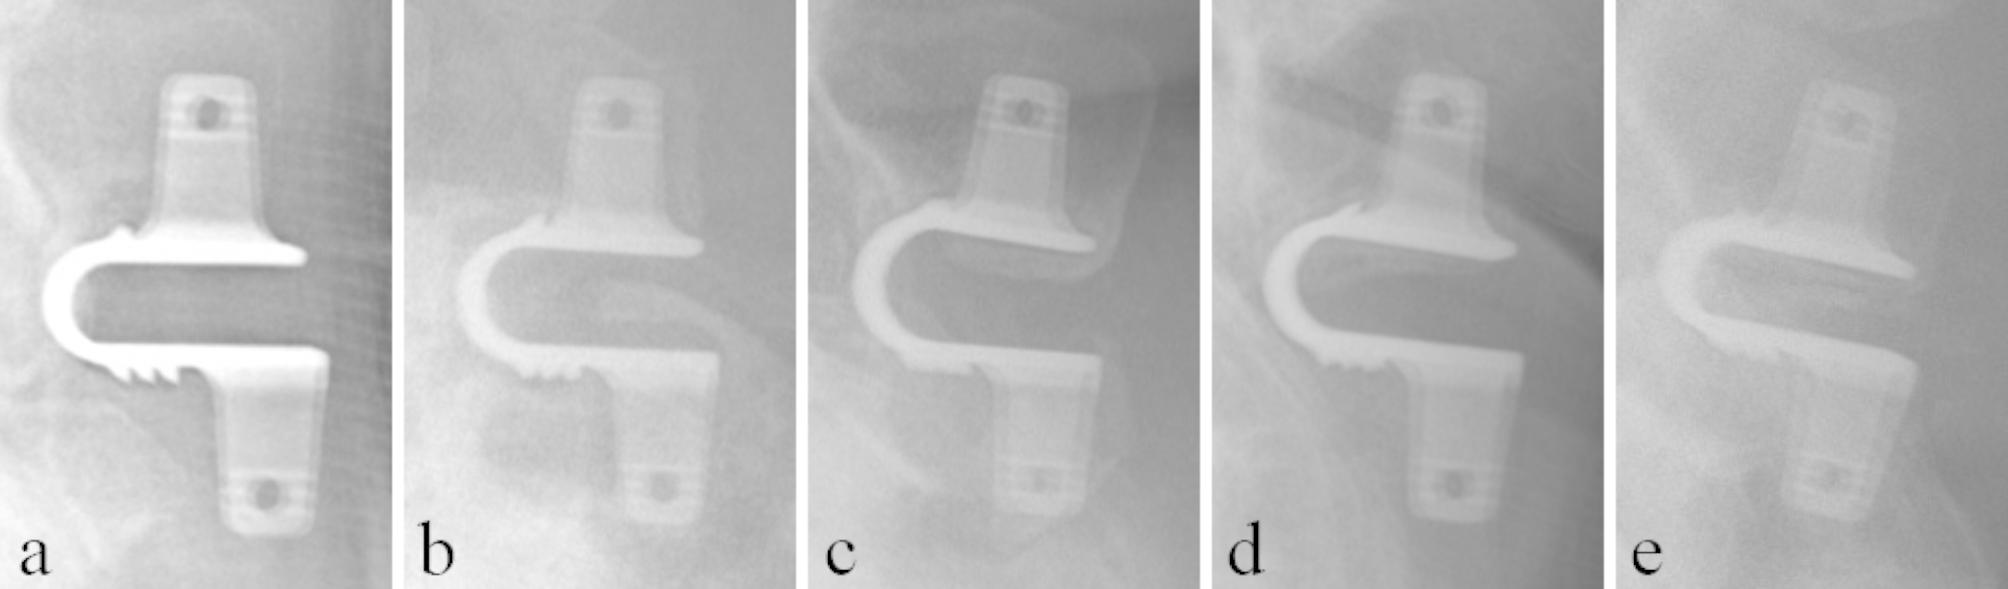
Radiographs of five patients, lateral views. a: No HO. b-e: Various forms of HO in the interspinous space
